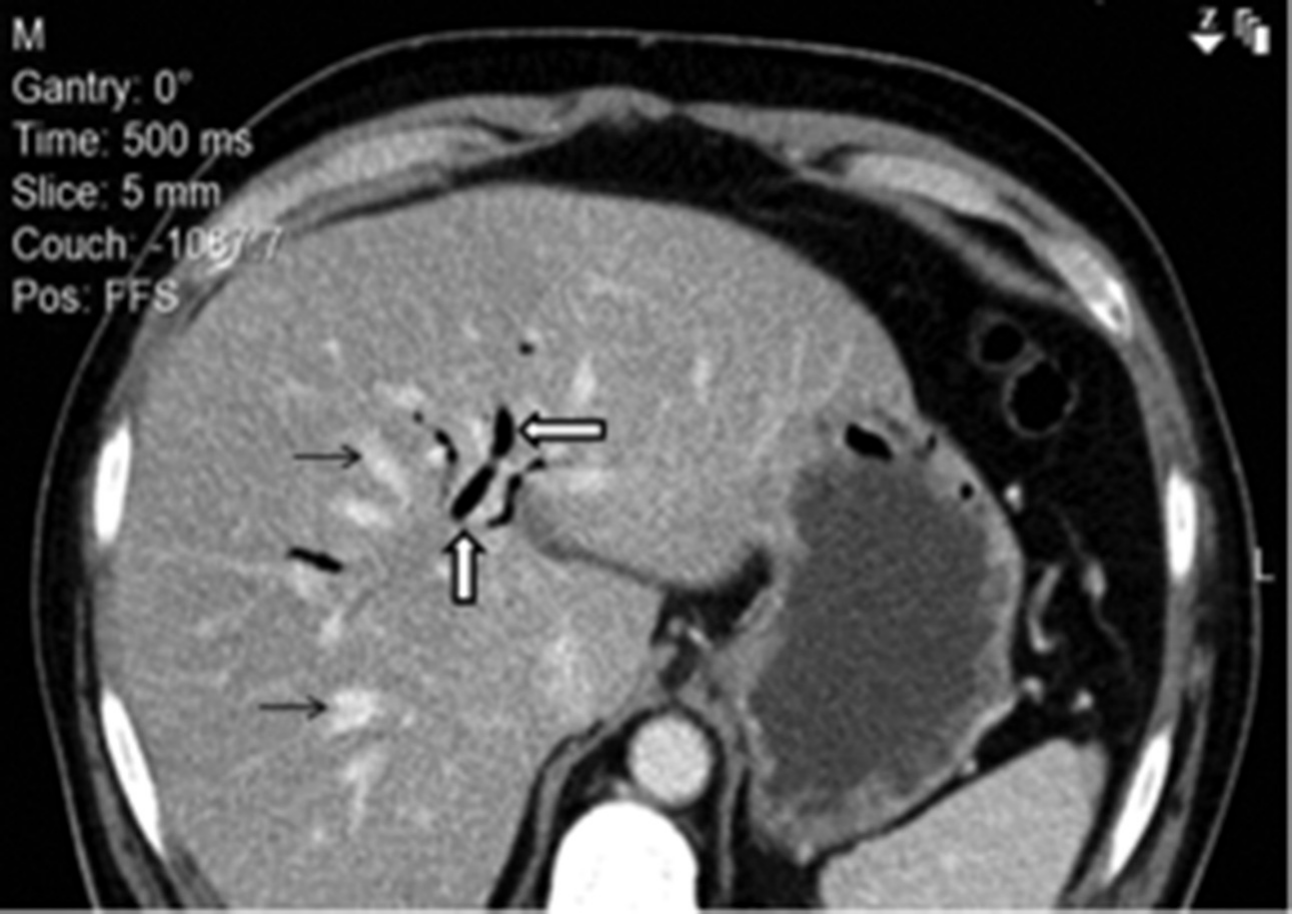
Axial contrast-enhanced computed tomography image of the patient’s abdomen revealing pneumobilia (thick white arrows) and dilated intrahepatic biliary radicles (thin black arrows).
